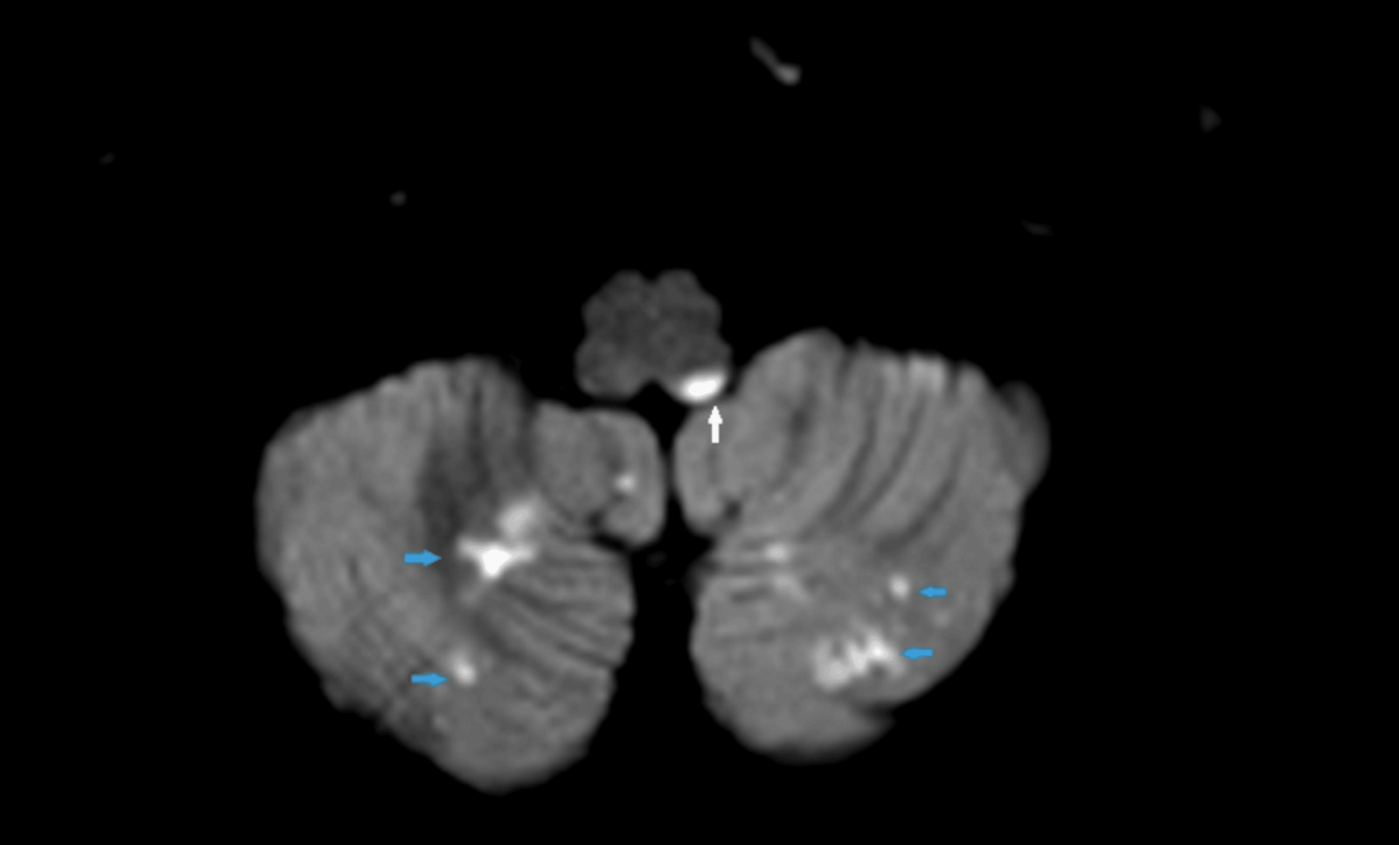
Repeat axial DWI magnetic resonance imaging of the brain performed 12 days after initial imaging shows progression of the cerebrovascular disease despite dual antiplatelet and statin therapy. There is enlargement of the left posterolateral medullary infarct with new acute infarcts identified in the left lateral cervico-medullary junction and left inferior cerebellum. DWI: Diffusion-weighted imaging
